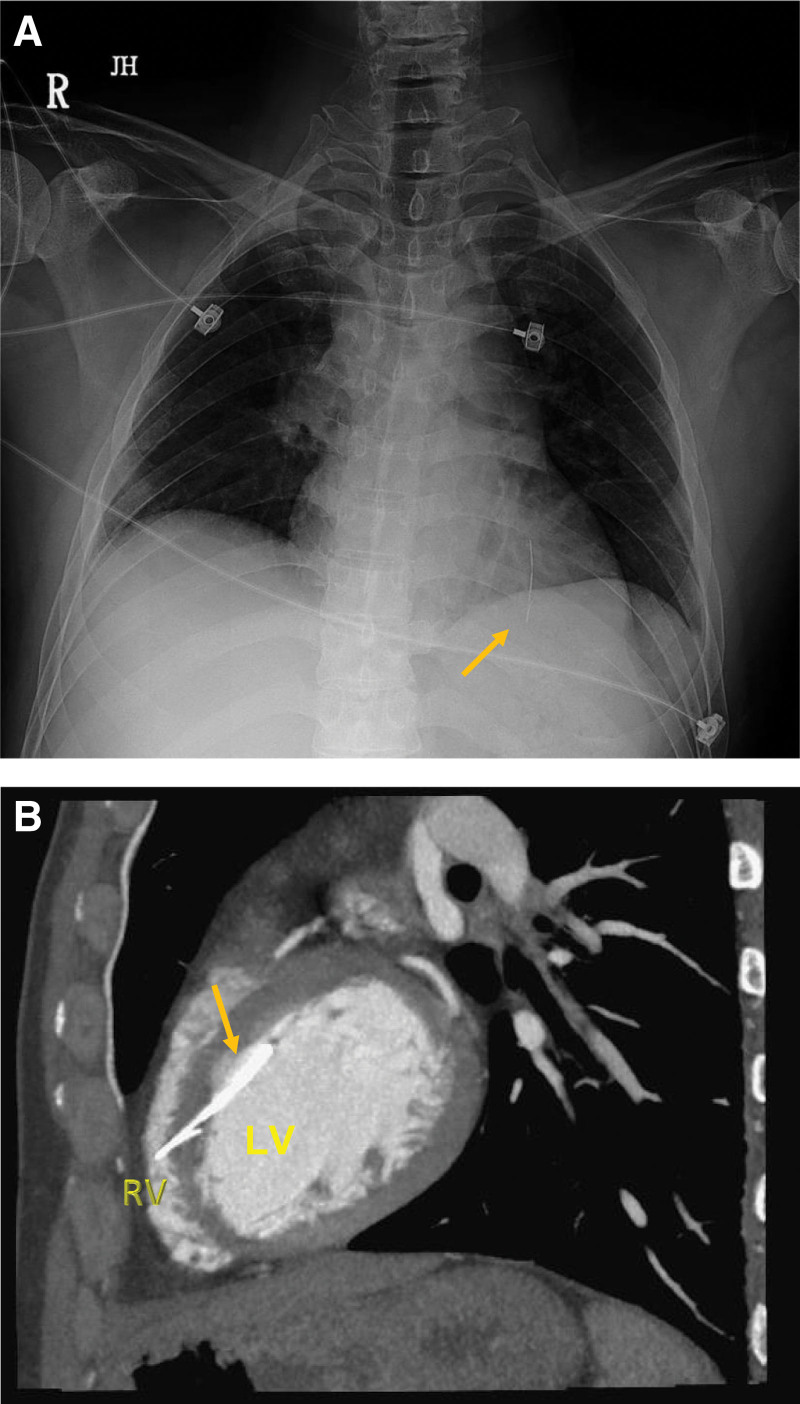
(A) A chest radiograph on the day of admission reveals a linear radiopaque density superimposed in the lower mediastinum. This is suggestive of a foreign body in the left ventricle. (B) Preoperative multi-detector computed tomography reveals a metallic foreign body in the left ventricle that penetrated the interventricular septum.
